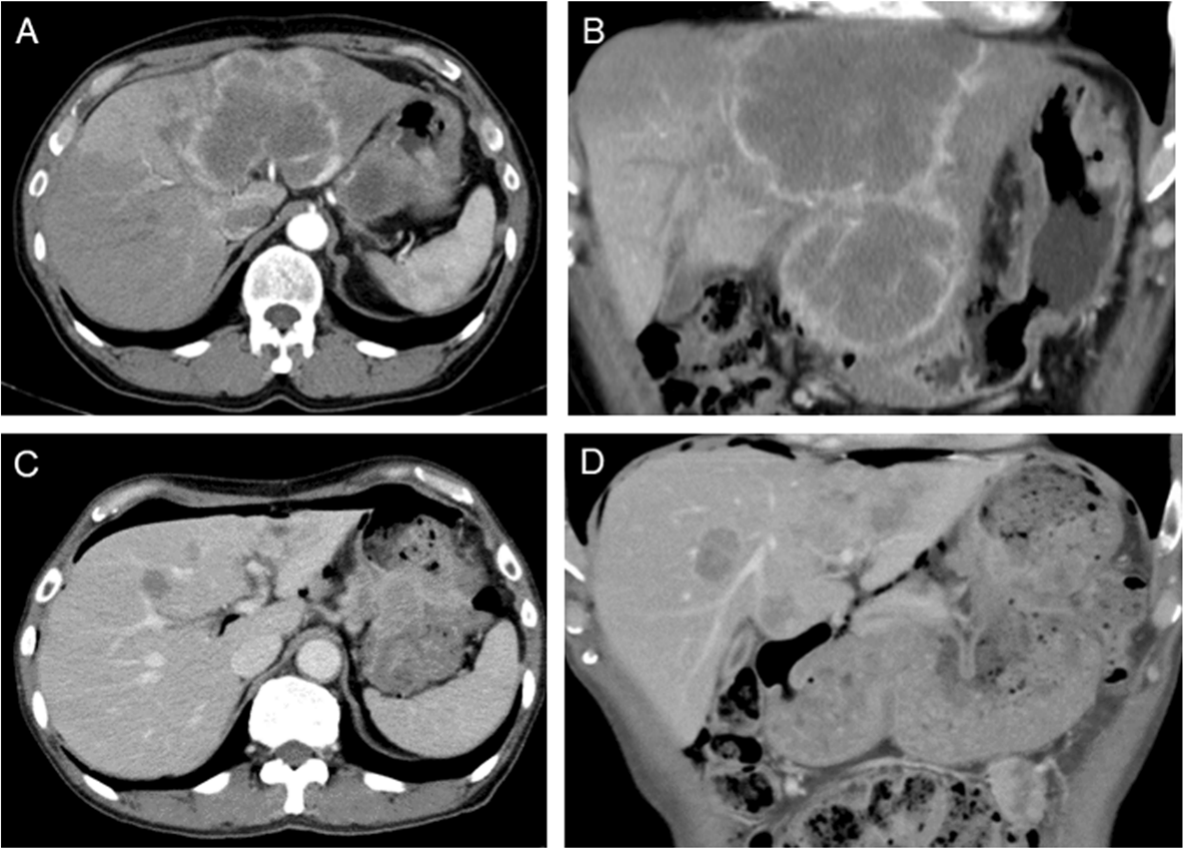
Computed tomography findings. Imaging before chemotherapy revealed thickening in the wall of the stomach and ring-enhanced liver metastases (100 mm and 105 mm in diameter). a Axial. b Coronal. Imaging after chemotherapy revealed that the stomach tumor and liver metastases had shrunken considerably. c Axial. d Coronal
